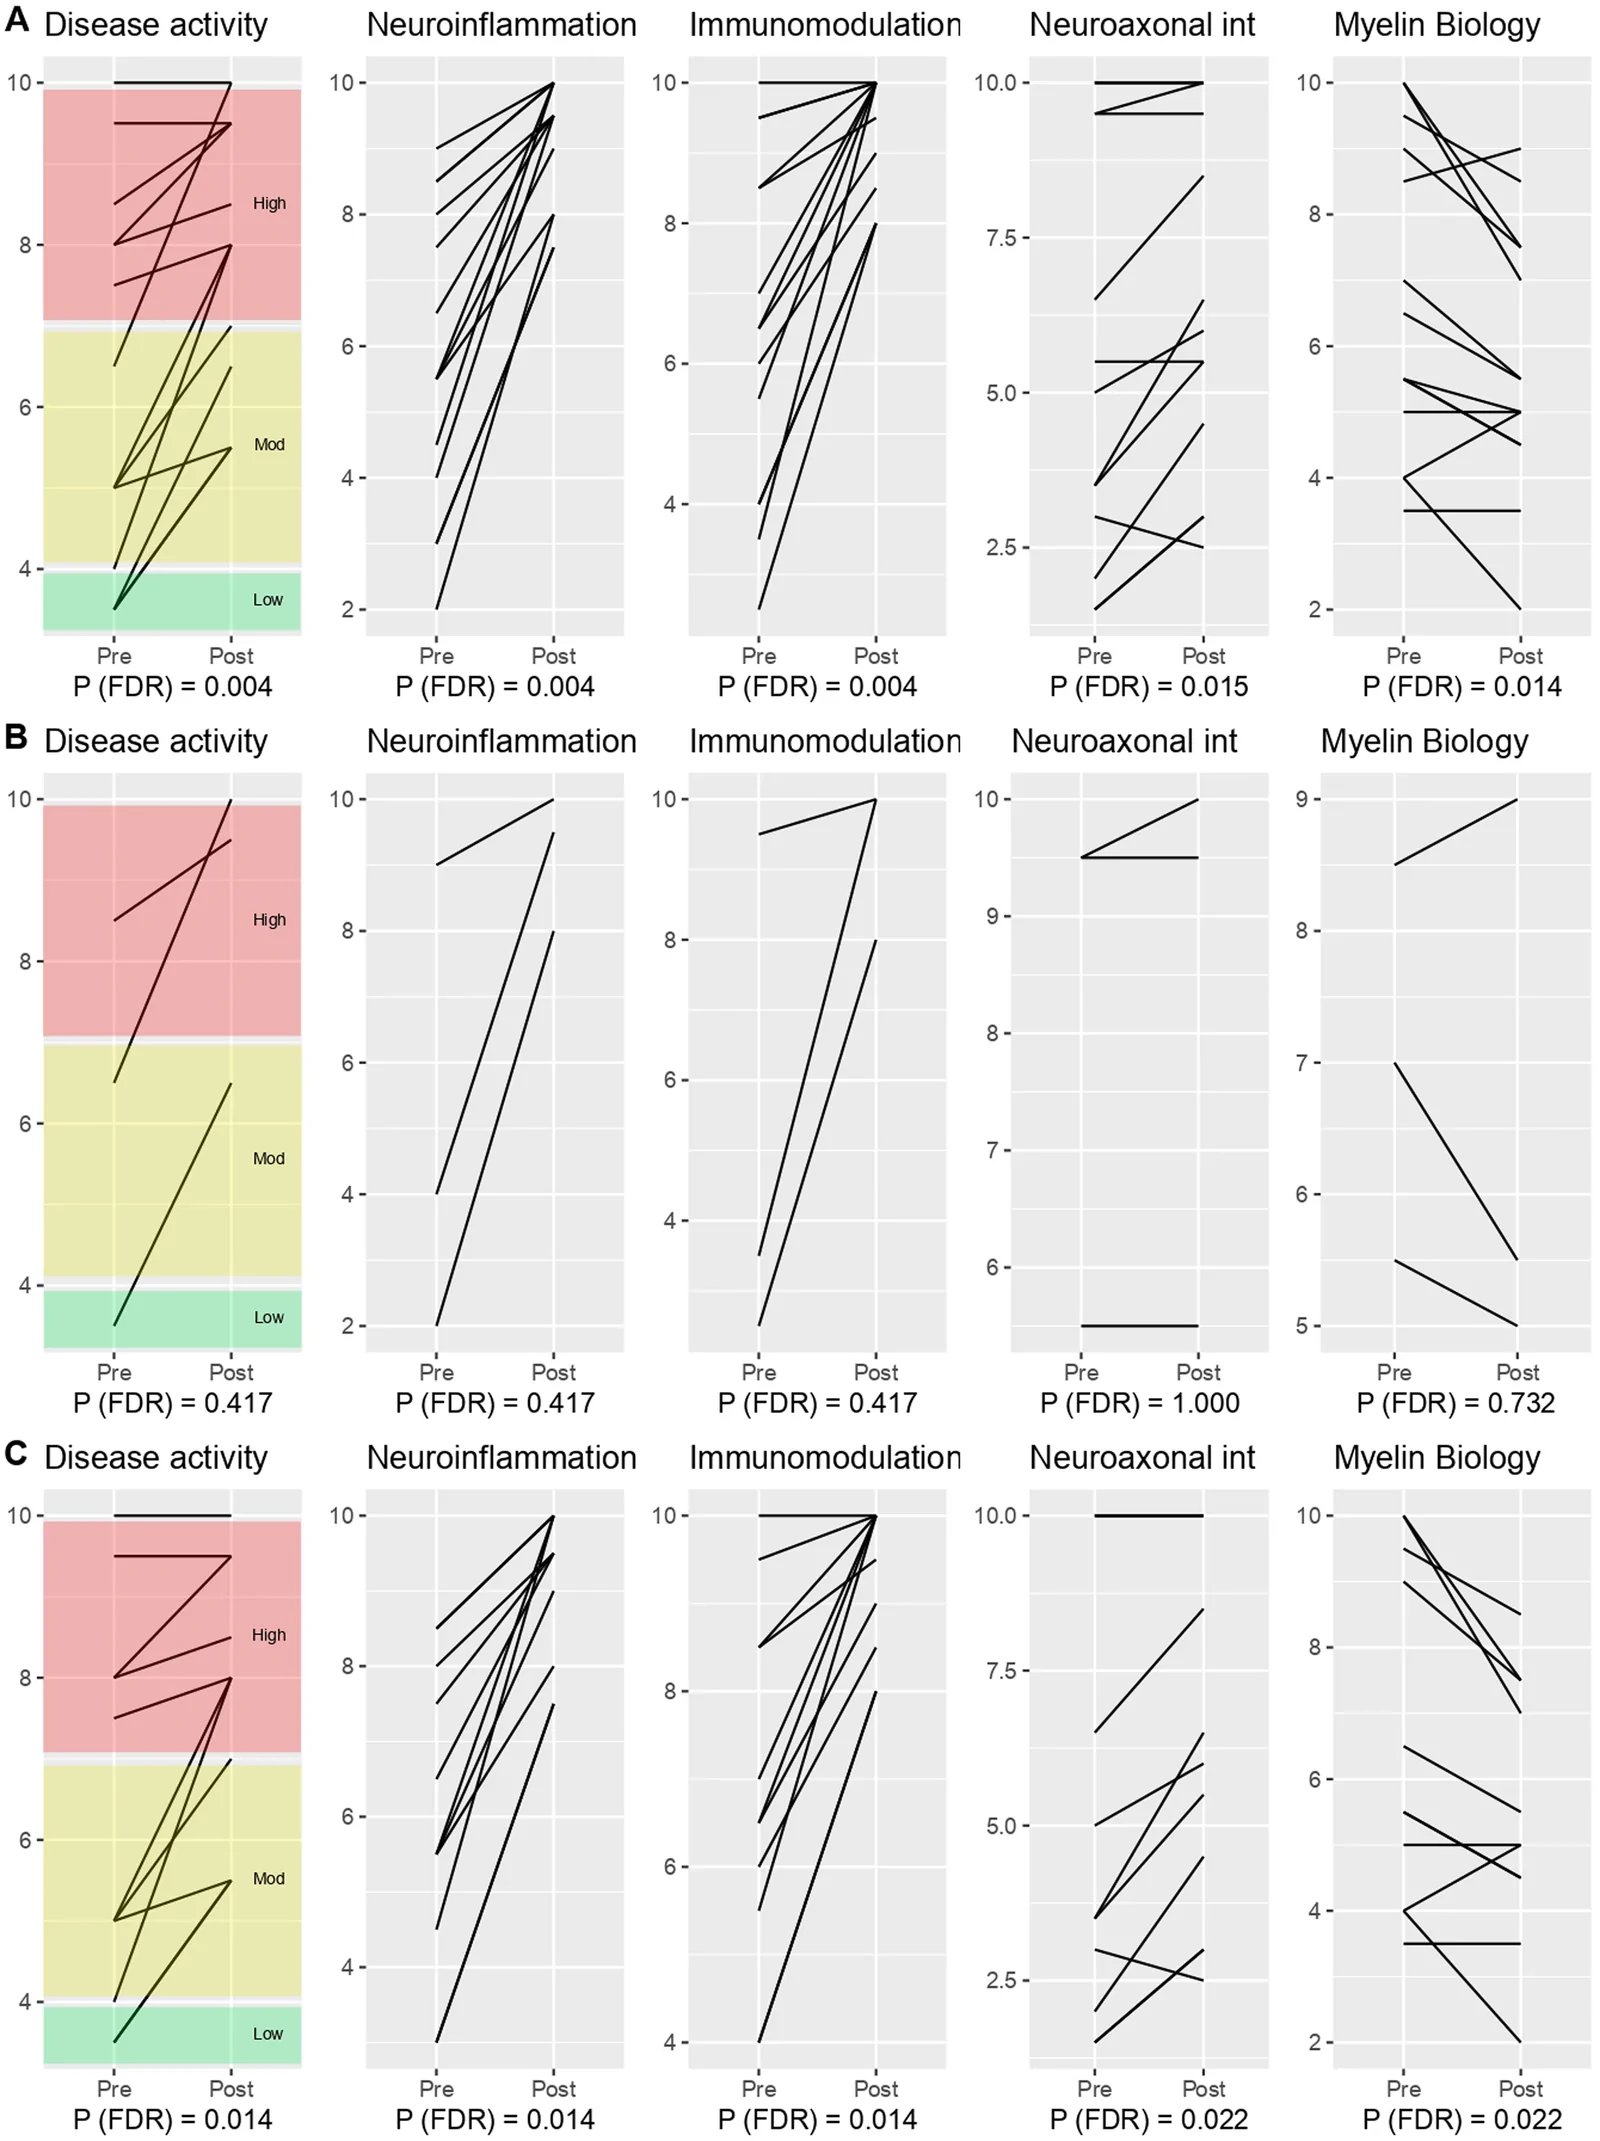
A 3-day course of high-dose IVMP causes significant change in Octave MSDA disease activity scores in (A) all patients with acute relapses of RMS; (B) patients with RMS without evidence of inflammatory activity on MRI; (C) patient with RMS with new gadolinium-enhancing lesions on MRI. Paired Wilcox rank-sum test was used to assess statistical significance and *p*-value for each test is provided below each graph. Disease Activity score levels (high, moderate, and low) are marked on each graph according to validated clinically meaningful categories.
